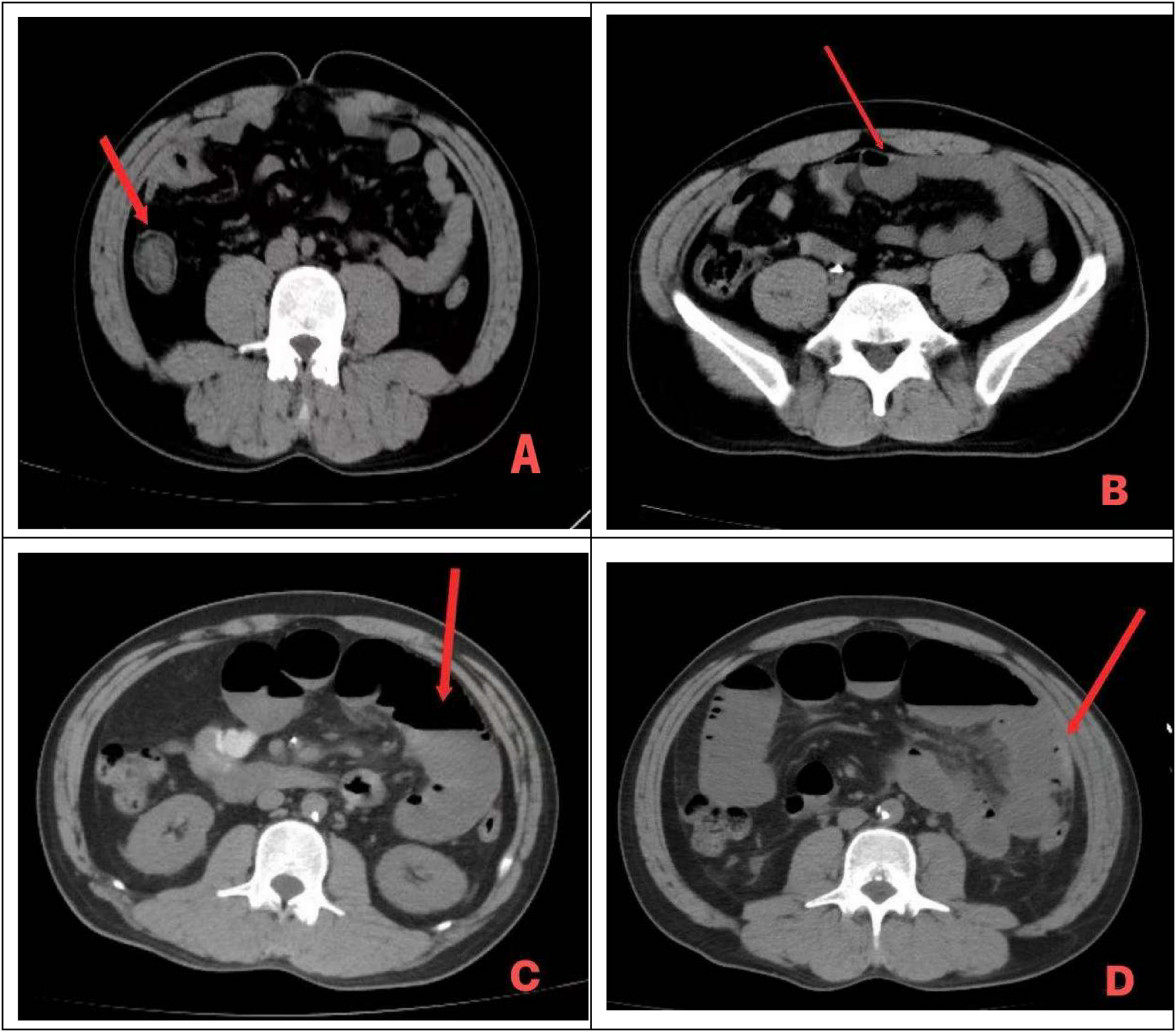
Non-contrast abdominal CT findings of the patient. (A) Abdominal CT on admission (8 December 2024) showing thickened and irregular bowel walls. (B) Follow-up CT on postoperative day 1 after endovascular intervention (11 December 2024) demonstrating mild dilation of some small bowel loops with fluid accumulation. (C) Follow-up CT on postoperative day 2 after endovascular intervention (12 December 2024) showing marked dilation of some small bowel loops with fluid accumulation and multiple air–fluid levels. (D) Follow-up CT on postoperative day 3 after endovascular intervention (13 December 2024) demonstrating dilation of the left-sided small bowel with gas and fluid accumulation, accompanied by surrounding exudation and a small amount of hemoperitoneum in the abdominopelvic cavity.
